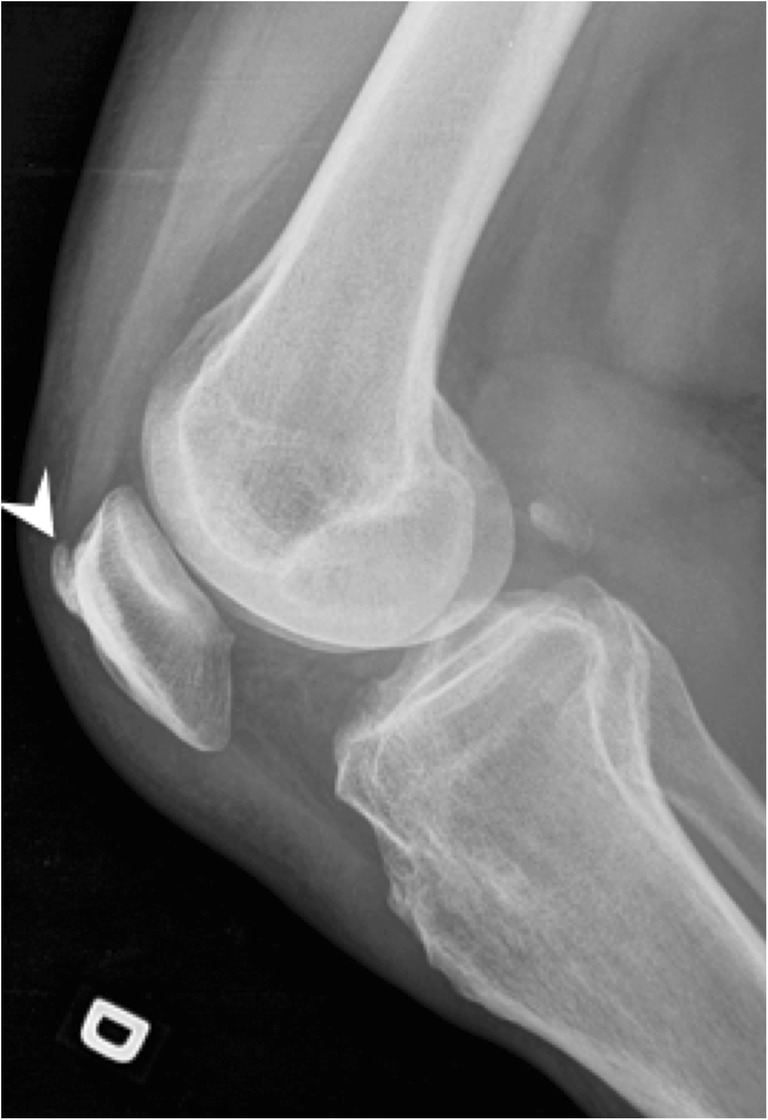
Enthesophyte. Lateral radiograph of the right knee shows an ossified enthesophyte at the insertion of the quadriceps tendon on the patella (arrowhead)
